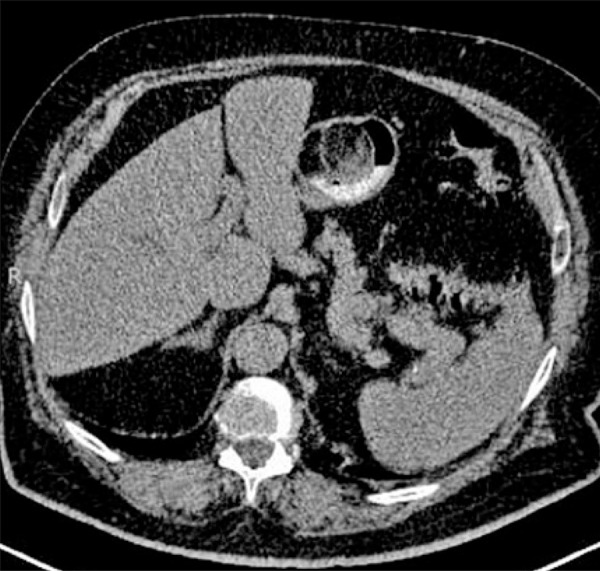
Computed tomography showing a well circumscribed mass with soft-tissue density and protruding into the gastric lumen
